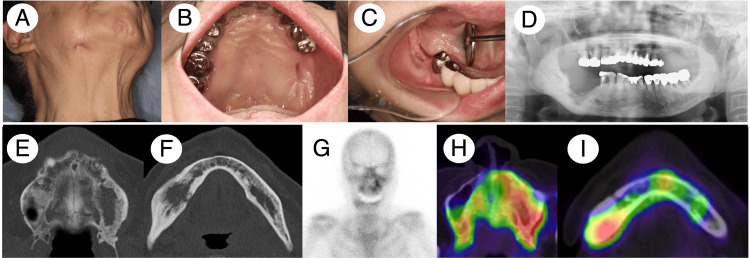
Patient 1 clinical and radiographic findings after the treatment. Extra- and intraoral examination unveiled the remaining cavity covered with granulation tissue or normal gingiva (A, B, and C). Panoramic X-ray and MDCT identified the signs of bone regeneration in the affected areas with periosteal reaction disappearance (D, E, and F). Bone scintigraphy and SPECT showed an apparent reduction of the 99mTc-MDP/HMDP accumulation in the affected areas (G, H, and I). MDCT, multidetector computed tomography; SPECT, single-photon emission computed tomography; 99mTc, technetium-99m; MDP, methylene diphosphonate; HMDP, hydroxy-methylene diphosphonate
